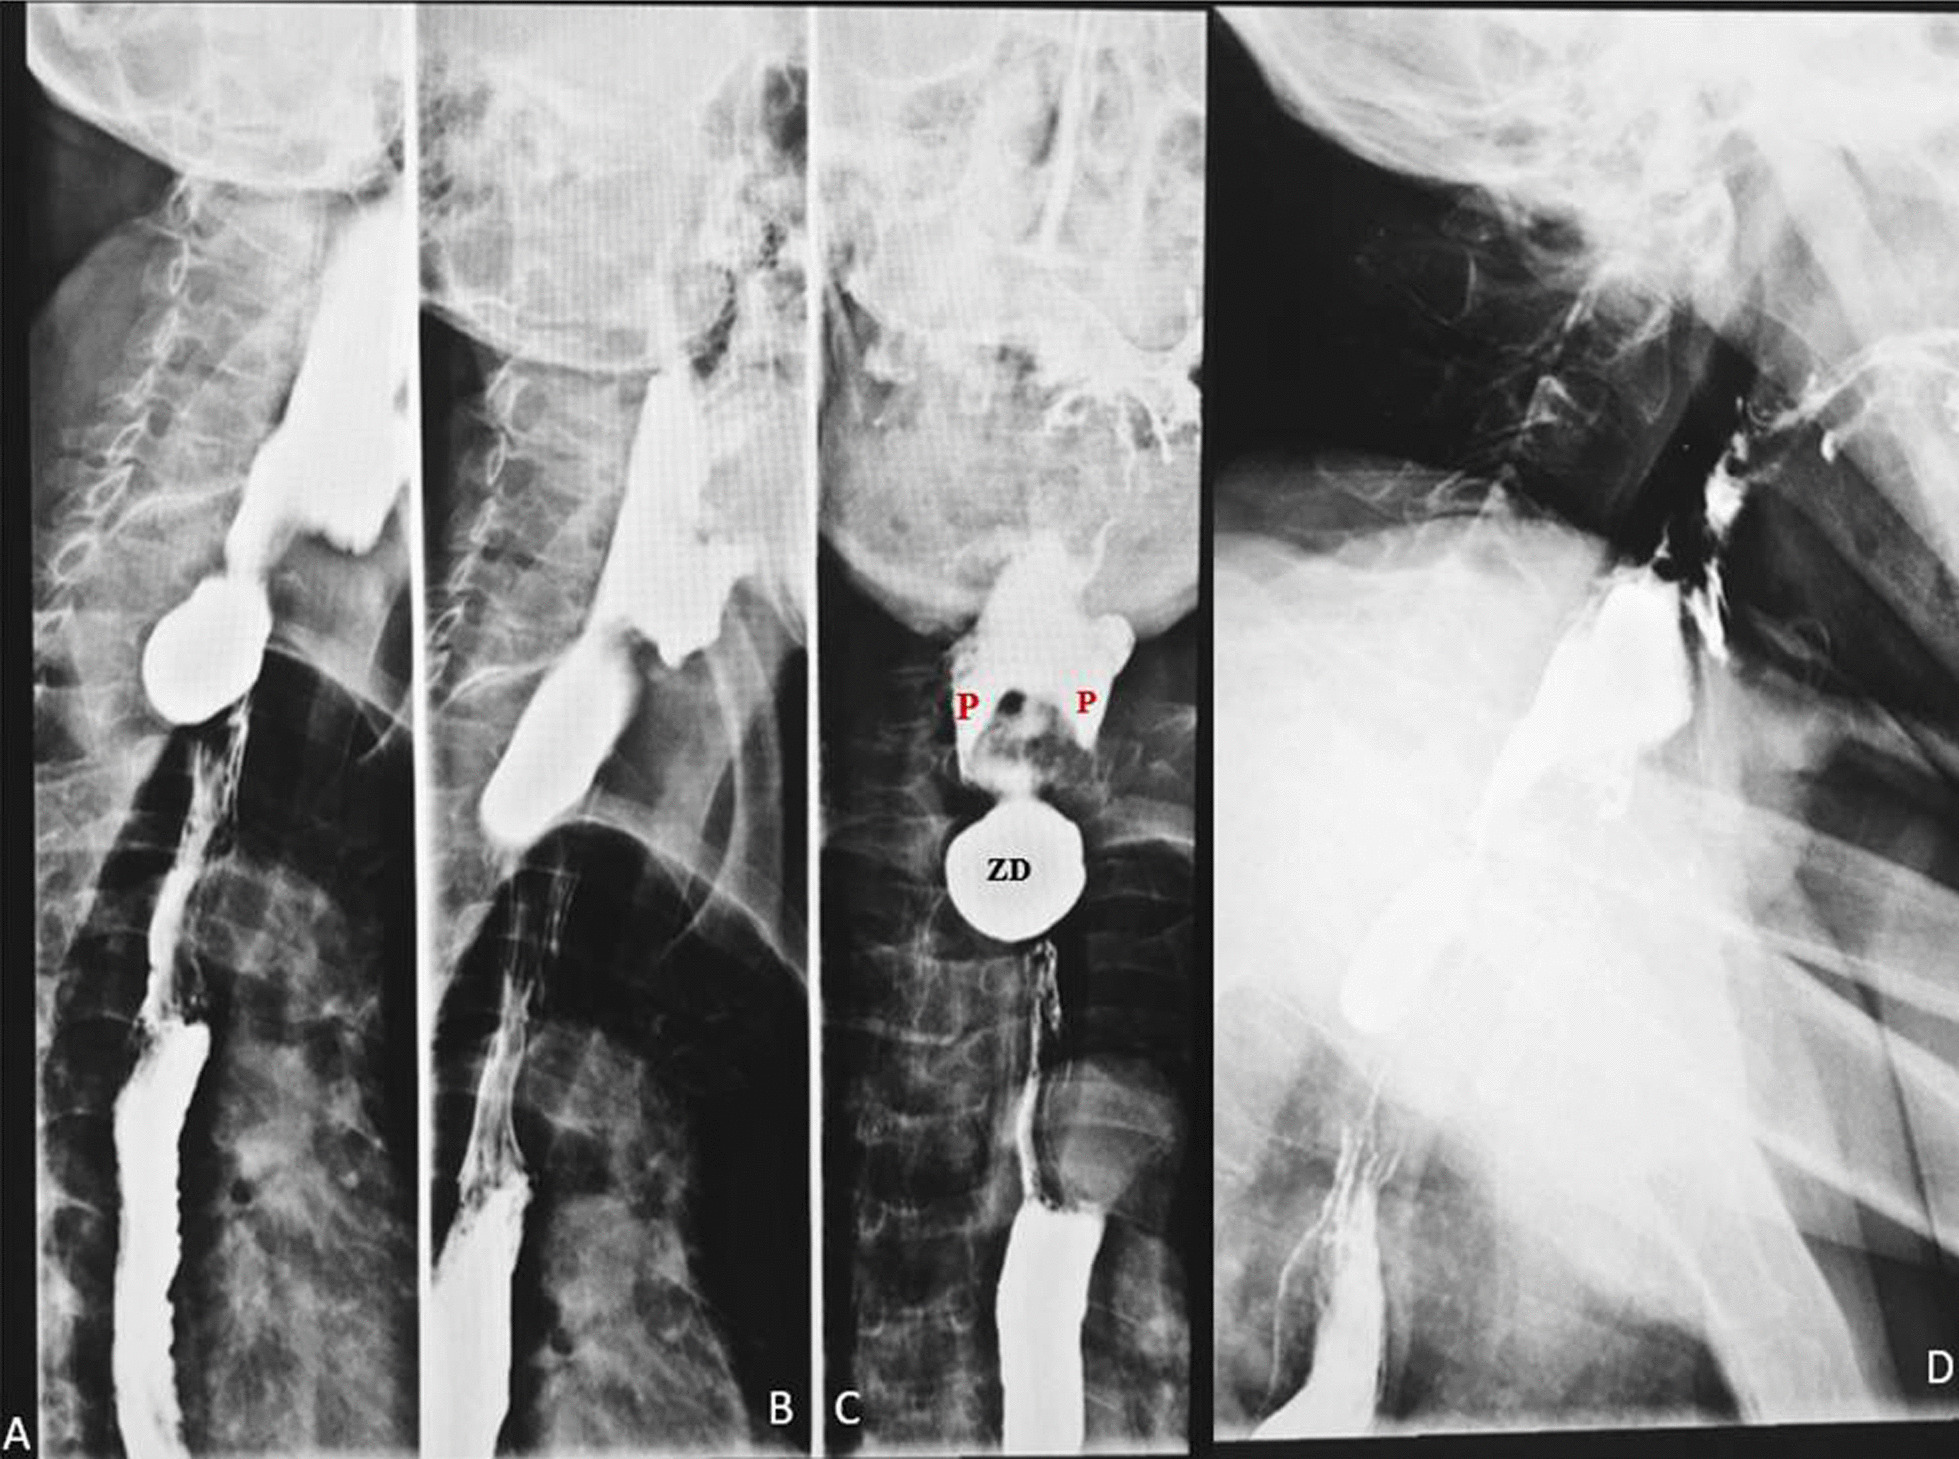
Static radiographic images taken from Videofluoroscopic esophagram A RAO view demonstrates posterior oval outpouching of the proximal esophagus with normal contrast flow in to the distal esophagus. B Between RAO and right lateral view demonstrated an elongation of the outpouching with additional contrast. C AP view demonstrated the dilated pyriform recess (P) and the diverticulum (ZD) D Lateral view demonstrates contrast coated pharynx and hypopharynx, pyriform and vallecullae
